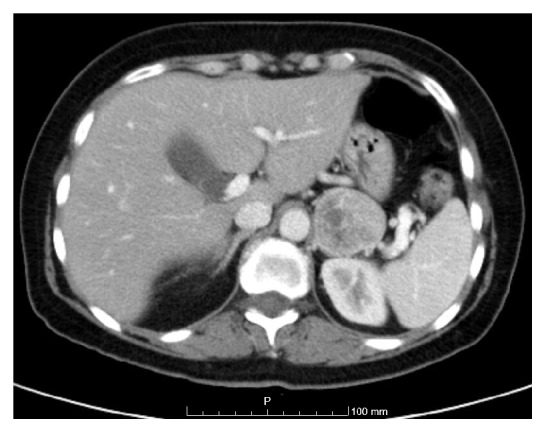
Adrenal tumor on abdominal CT scan*. Adrenal tumor with 4.2cm length and heterogeneous iodine contrast uptake.
